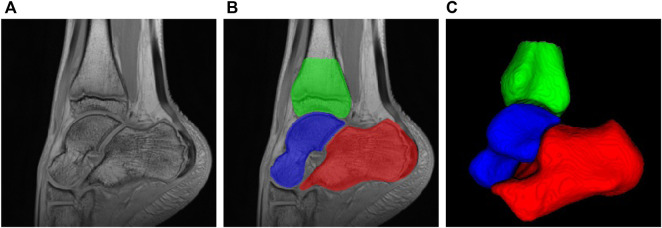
MRI of ankle joint and bone of interest: (A) Sagittal view of ankle static 3D MRI; (B) Segmentation of bone of interest: red: calcaneus, blue: talus, green: tibia; (C) 3D rendering of the three bones of interest.
